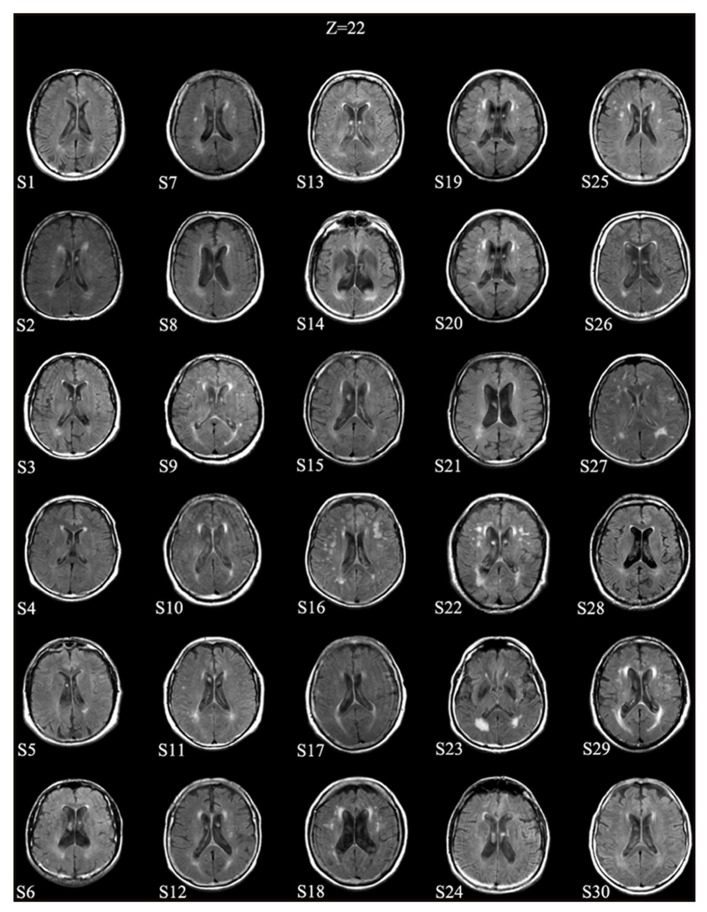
Patients’ MRI scans. A representative axial slice (at approximate MNI *Z*-level of 22) of FLAIR images for each patient is shown. Images are in neurological convention (left is left).
